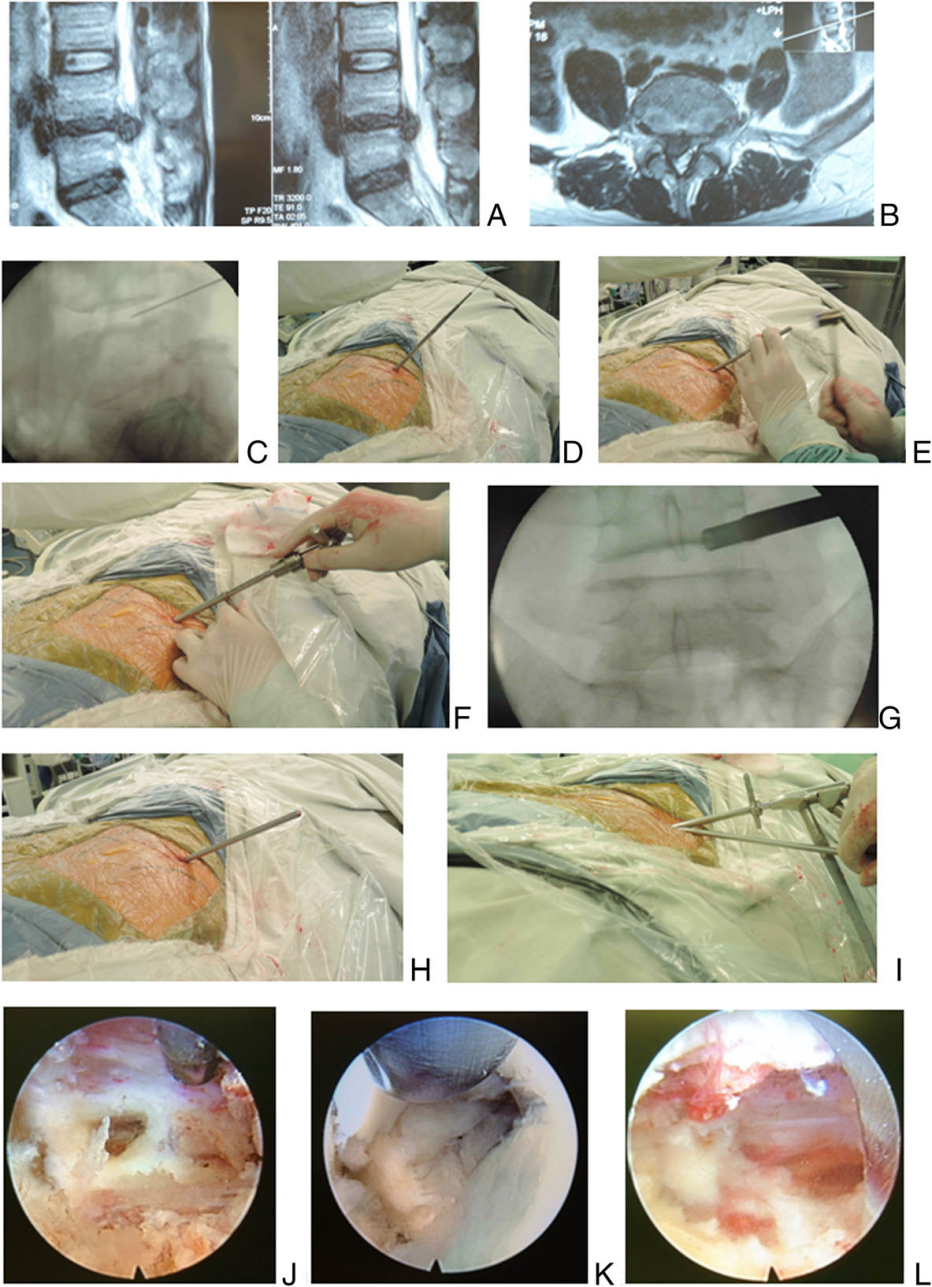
Male patient (47 years) of L4/5 disc herniation with extruding fragment underwent the procedure of PTES. a Sagittal and b axial MR images showed L4/5 disc herniation. During the procedure of PTES, The puncture needle was anteromedially inserted at about 45° angle to the horizontal plane and the tip of puncture needle was in the posterior one third intervertebral space on c lateral C-arm view. Over the guiding wire, stepwise-dilating cannulas were introduced to annulus fibrosus through the foramen d. The thick guiding rod was advanced with a mallet into the foramen after removal of the guiding wire e. An 8.8-mm cannula was pushed over the rod to the facet joint area, docked at the superior facet after the rod was  removed, and pressed down to make the angle of the cannula to the horizontal plane smaller. Through this cannula, a 7.5-mm hand reamer was then introduced to ream the ventral part of facet bone until the resistance faded (press-down enlargement of the foramen) f, which was checked with g posteoanterior C-arm image. The reamer was removed, and the thick guiding rod then was reintroduced and advanced with the aid of a mallet until the tip of the guiding rod was into the herniated fragment h. A 7.5-mm working cannula was advanced over the guiding rod directly to the extruding fragment and the spine endoscope was inserted i. Under j, k, and l endoscopic view, the extruding disc fragment could be observed and the freed nerve root could be visualized after the massive nucleus underneath the nerve root were removed
